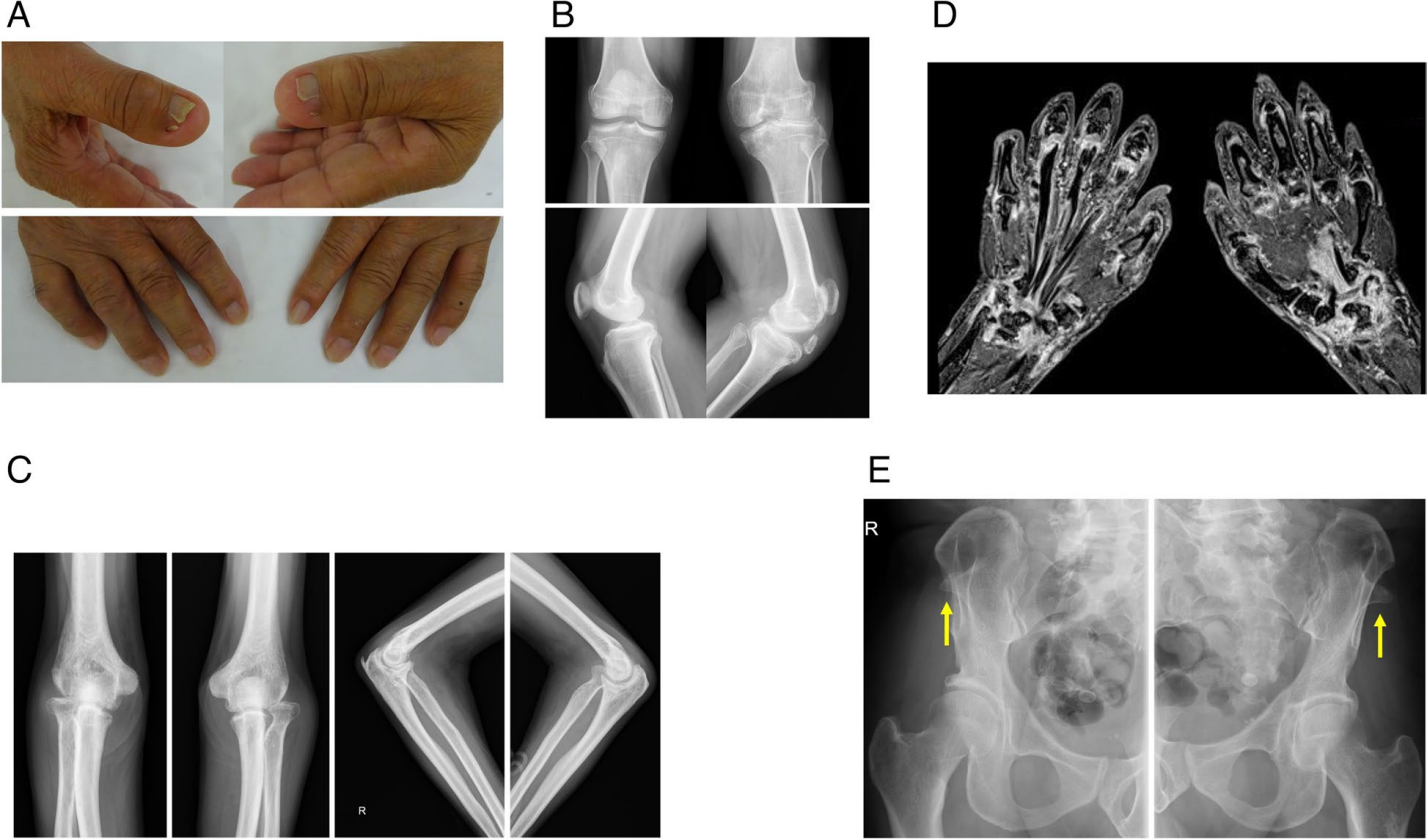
A Images of the patient’s nails. Dysplasia on the ulnar border of bilateral thumb nails (upper) and triangular lunules (lower). B X-ray of the knees showed mild hypoplasia of bilateral patellae and degeneration of the left knee. C X-ray of the elbows showed hypoplasia of the radial head and joint space narrowing in the right elbow. D MRI of the hands showed synovitis with synovial hyperplasia, bone marrow edema and erosion in bilateral wrists and in proximal interphalangeal and metacarpal phalangeal joints. E X-ray of the pelvis showed bilateral iliac horns (arrow)
